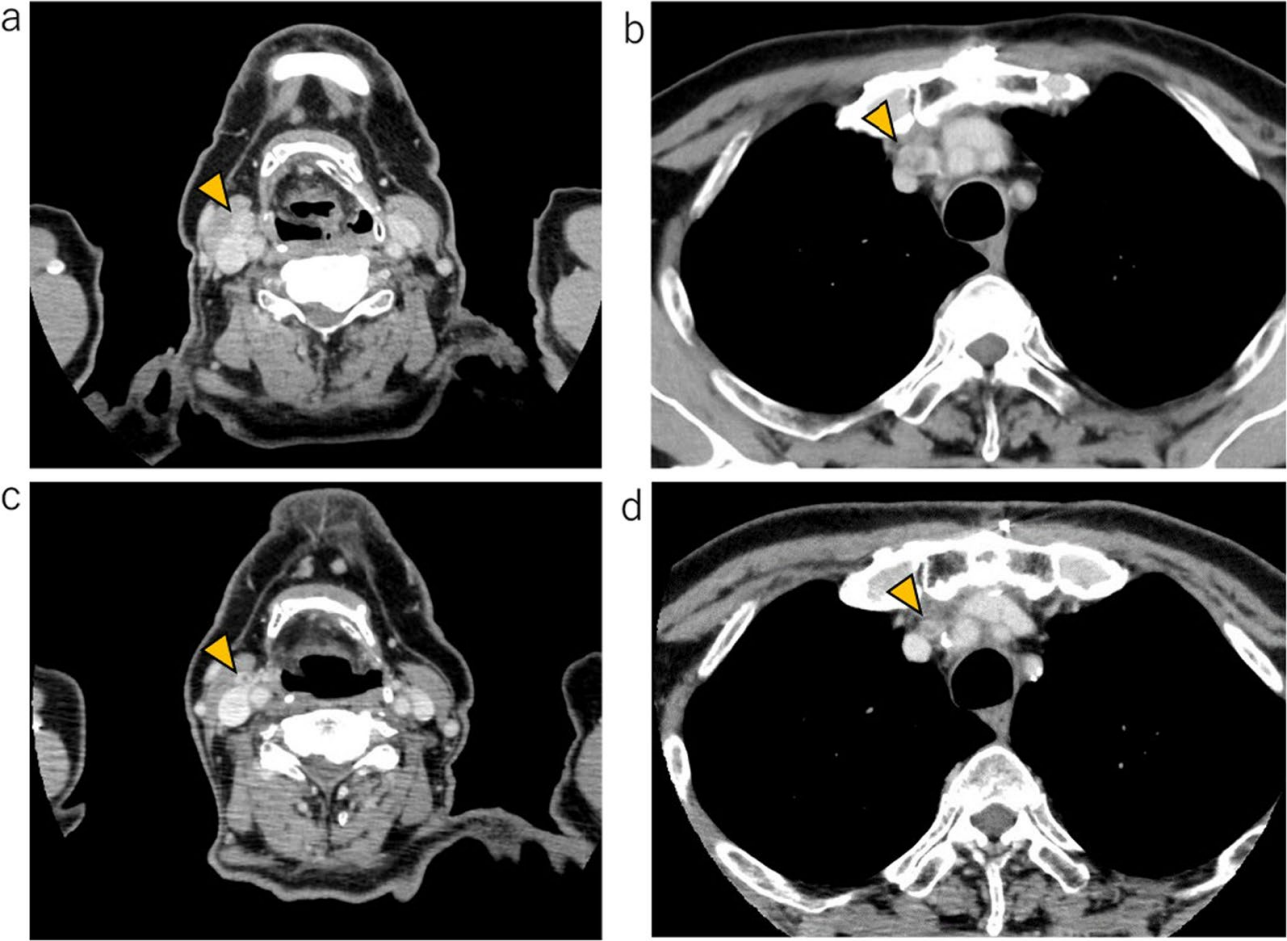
Computed tomography (CT) scans before and after selpercatinib treatment. CT scans at 11 months postoperatively revealed recurrence of mixed medullary and follicular cell-derived thyroid carcinoma at the right cervical lymph nodes (a) and upper mediastinal lymph nodes (b). After 9 weeks of treatment with selpercatinib, the cervical (c) and mediastinal lymph nodes (d) showed a reduction in size
